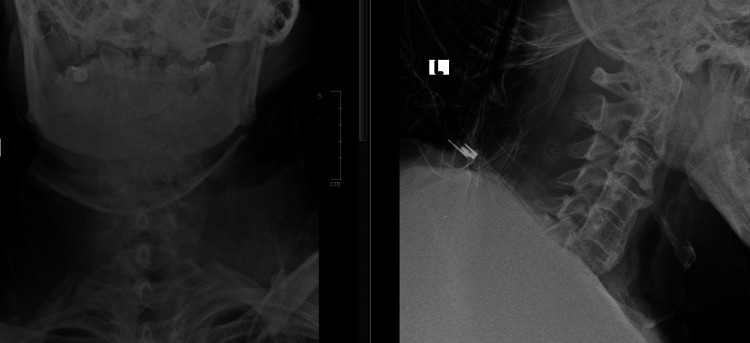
Anteroposterior and lateral view of plain cervical radiograph: no obvious fracture visible
